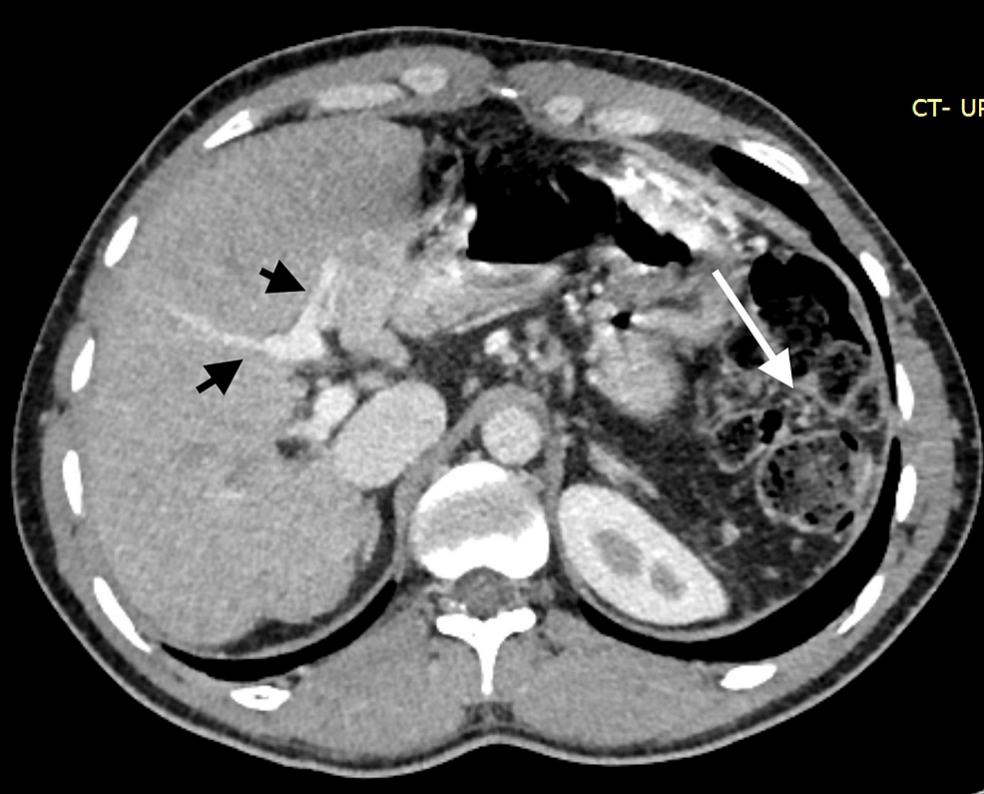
Axial abdominal CT after the shunt procedure. Axial CT scan showing normal liver parenchyma and a large left branch of the portal vein as compared to the right (arrowheads) and an absent spleen (arrow).
